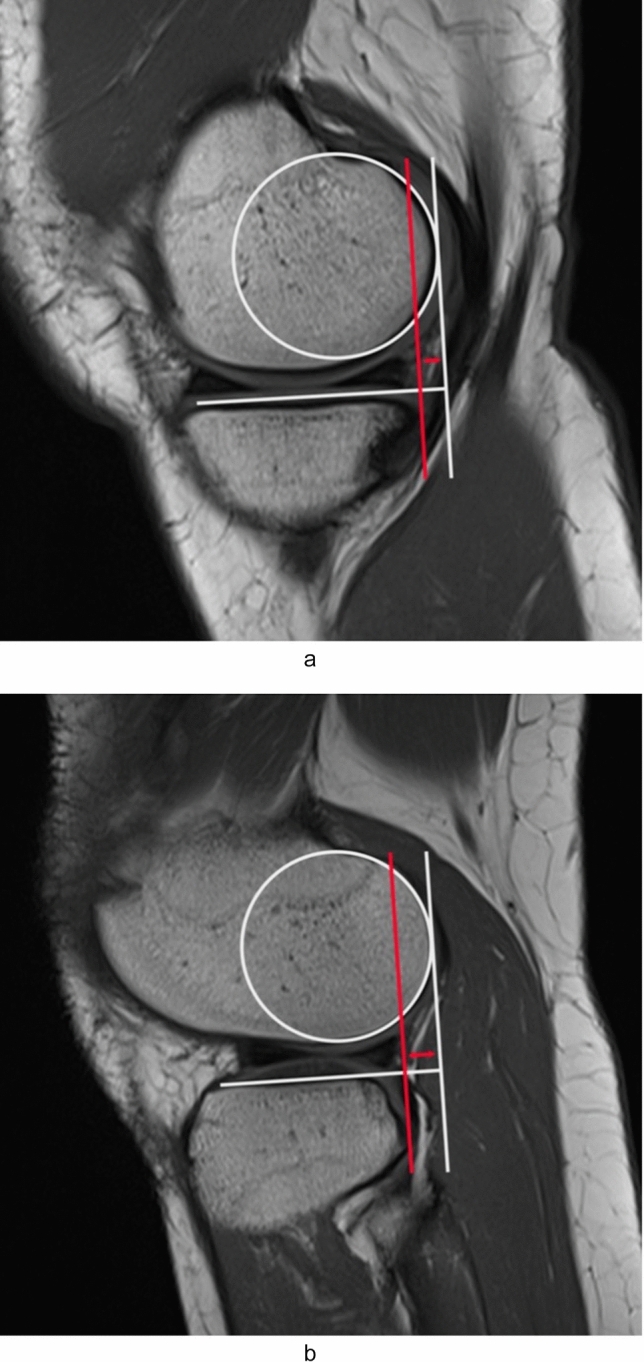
Assessment of anterior tibial translation (ATT) on sagittal T1-weighted knee MRI. A circle is drawn to fit the posterior femoral condyle. Two lines, perpendicular to the tibial plateau, are drawn: one tangent to the circle (white vertical line) and one tangent to the posterior tibial aspect (red line). The distance between these two lines represents the ATT. a Medial ATT, assessed at the level of the first image showing the origin of the medial gastrocnemius tendon. b Lateral ATT, calculated on the slice at the most medial aspect of the fibula at the tibiofibular joint
